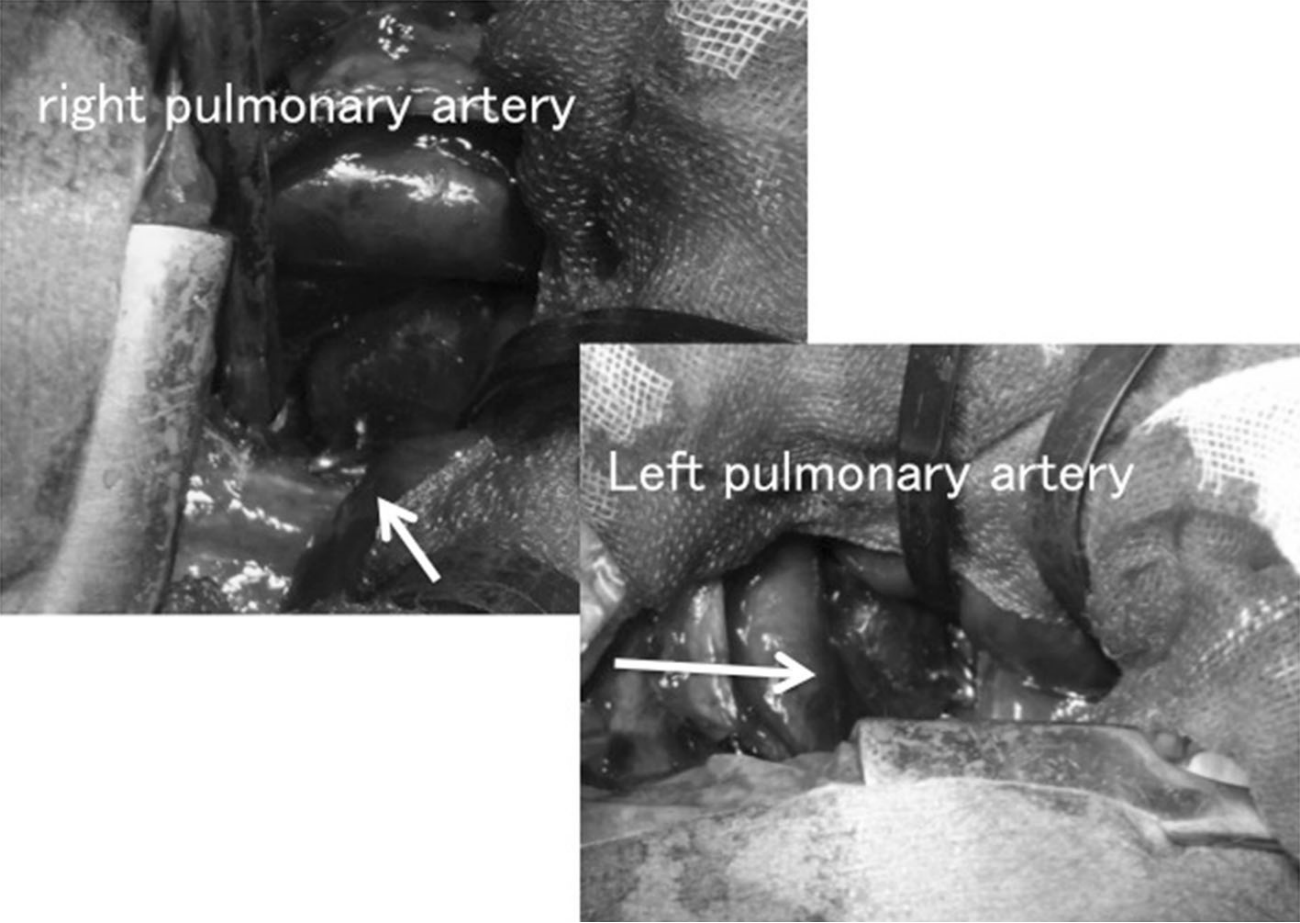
We used a hemoclip (*arrows*) as a partial clamp, instead of taping, to control the bleeding. Color Doppler showed a blood velocity of 3.0 m/s in the right PA banding site, suggesting that the banding was effective
